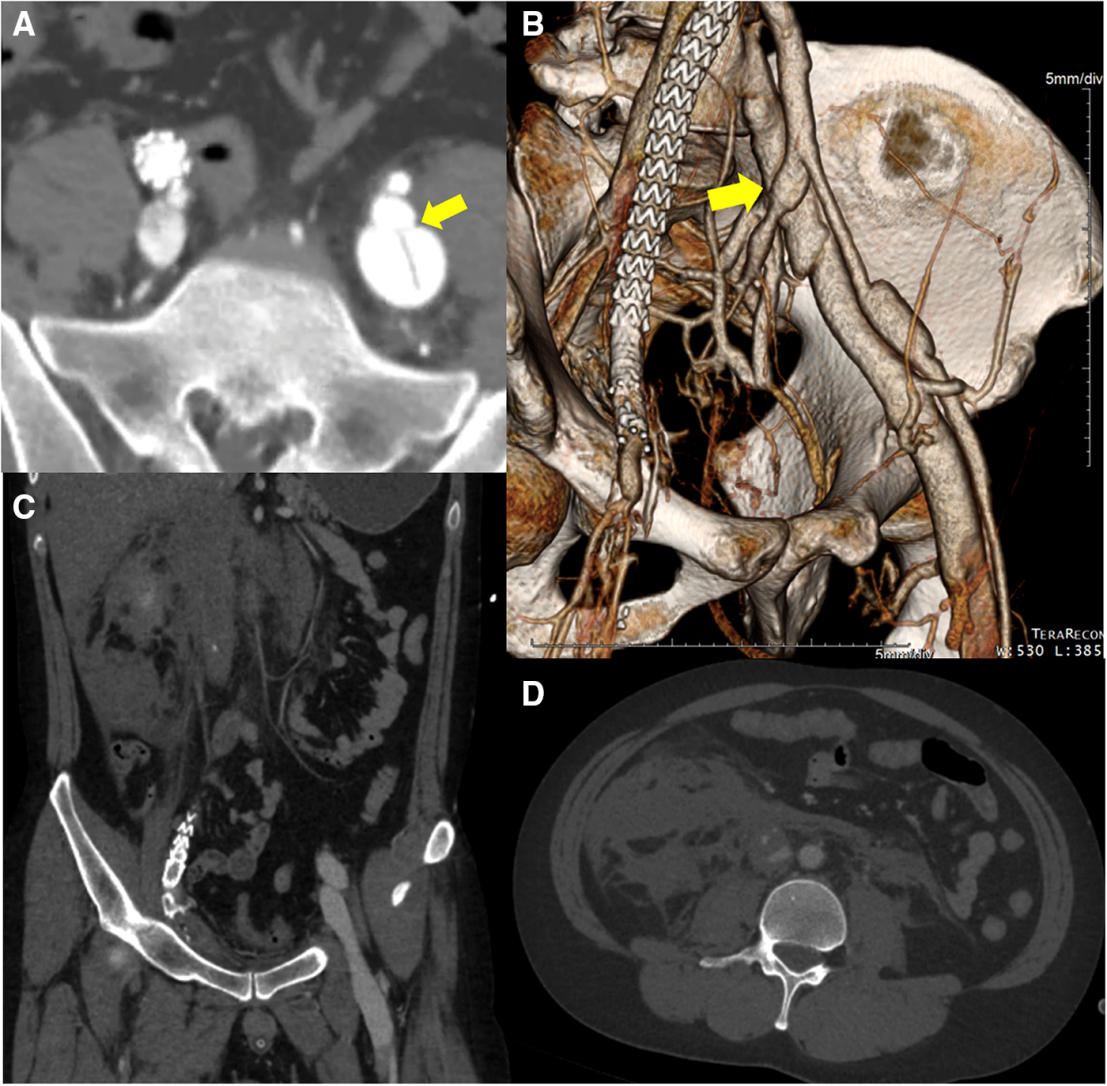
The initial computed tomography angiography before the 2nd operation reveals (A, B) an arteriovenous fistula (*yellow arrow*) between left internal iliac artery and left common iliac vein. Simultaneous contrast enhancement of arteries and veins is confirmed. (C, D) Large amount of hematoma near infrarenal inferior vena cava probably due to the intravenous high pressure generated by the iliac arteriovenous fistula and disease-specific vascular fragility.
